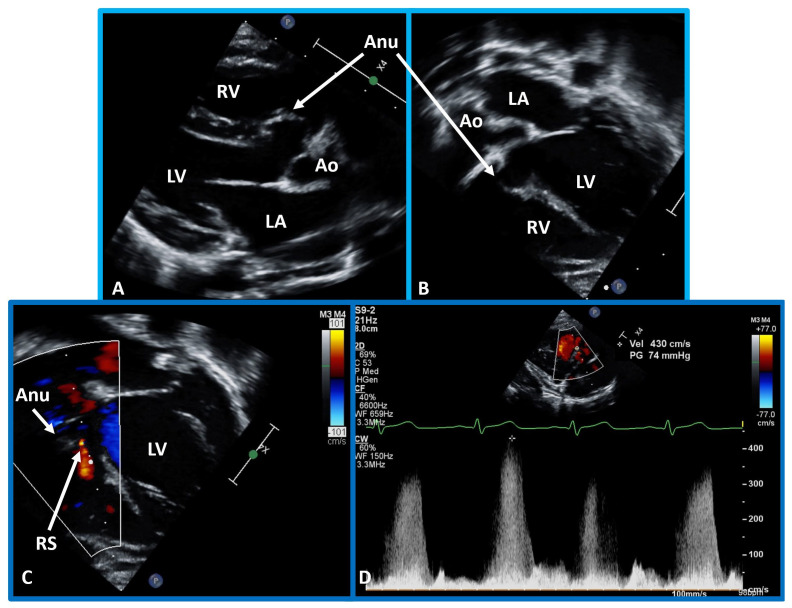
Echo-Doppler visualization of a perimembranous VSD. This figure
